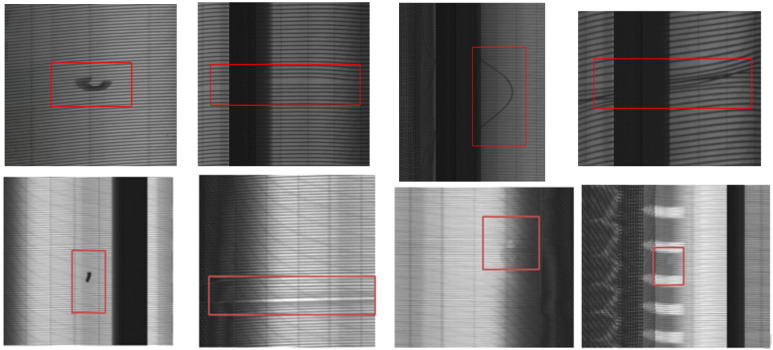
Typical defects in tire X-ray images.
